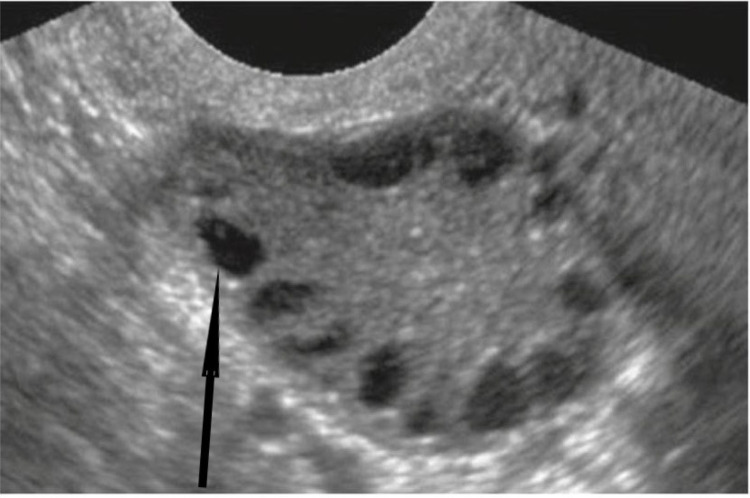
Pelvic ultrasound shows multiple ovarian follicles (arrows) A 14-year-old girl with congenital adrenal hyperplasia and poor hormonal control developed polycystic ovary syndrome (PCOS). Fourteen ovarian follicles fulfilling the PCOS diagnostic criteria are visible via pelvic ultrasound.
